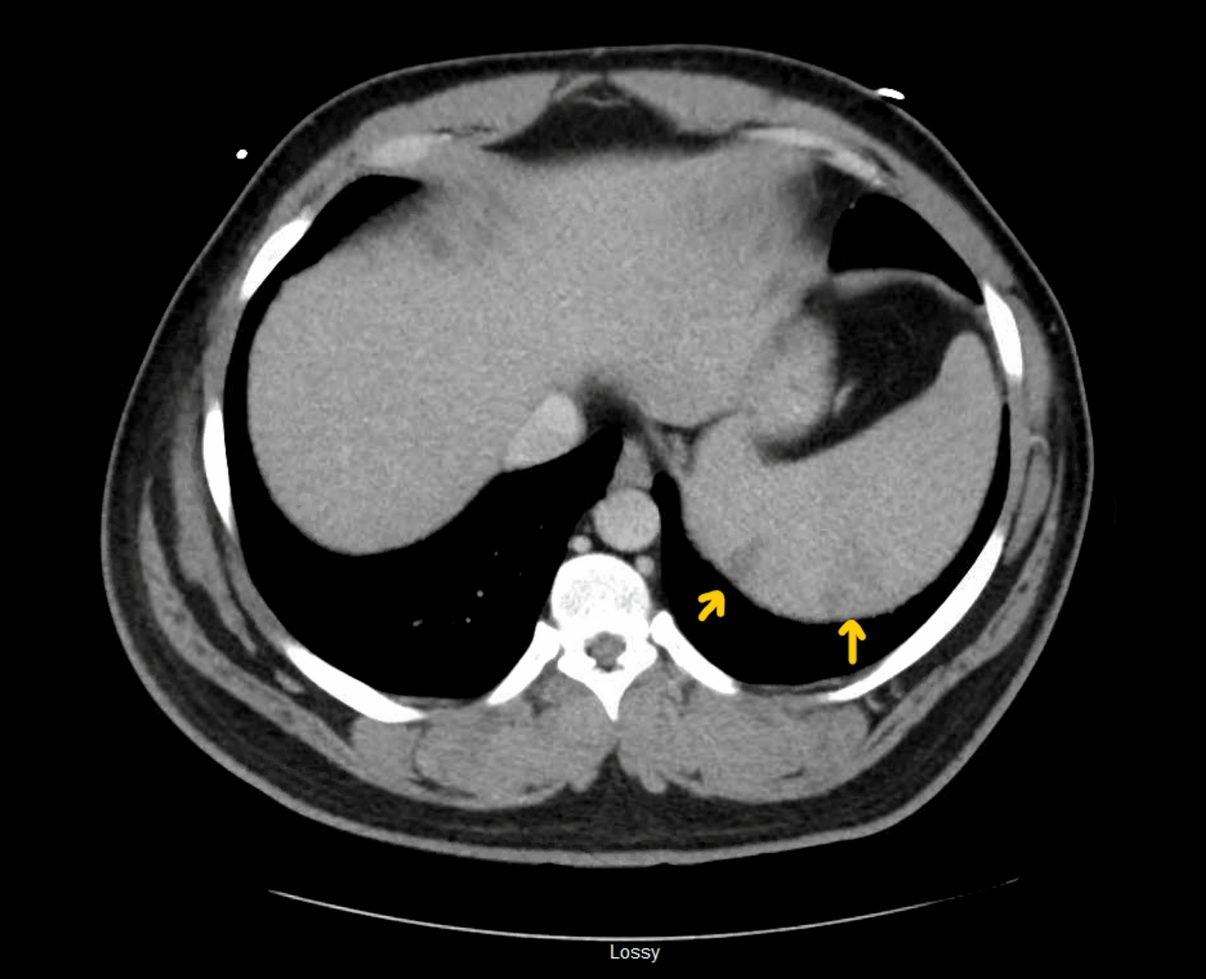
CT scan abdomen with IV contrast revealed splenomegaly with wedge-shaped infarcts
